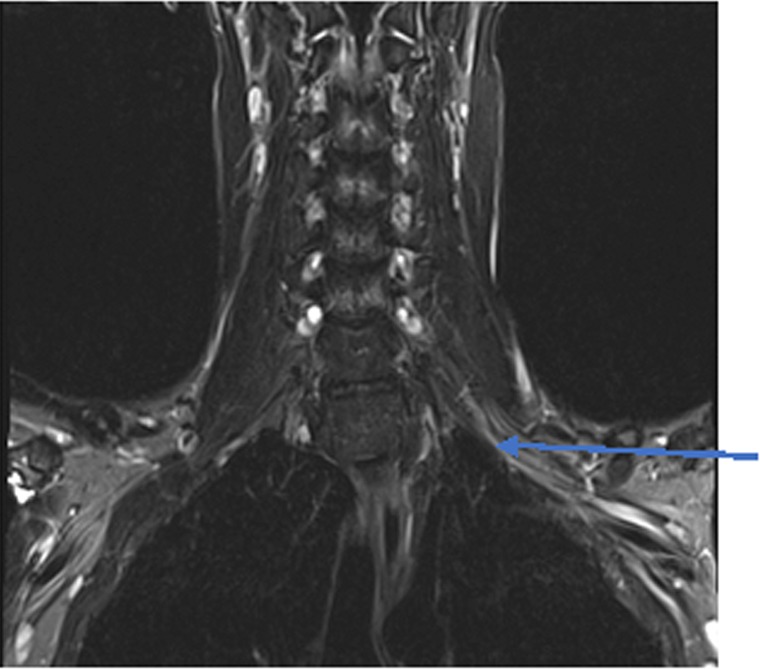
MRI brachial plexus with and without contrast. T2 coronal section shows patchy T2 hyperintensity of the left brachial plexus affecting all the trunks (arrow).
